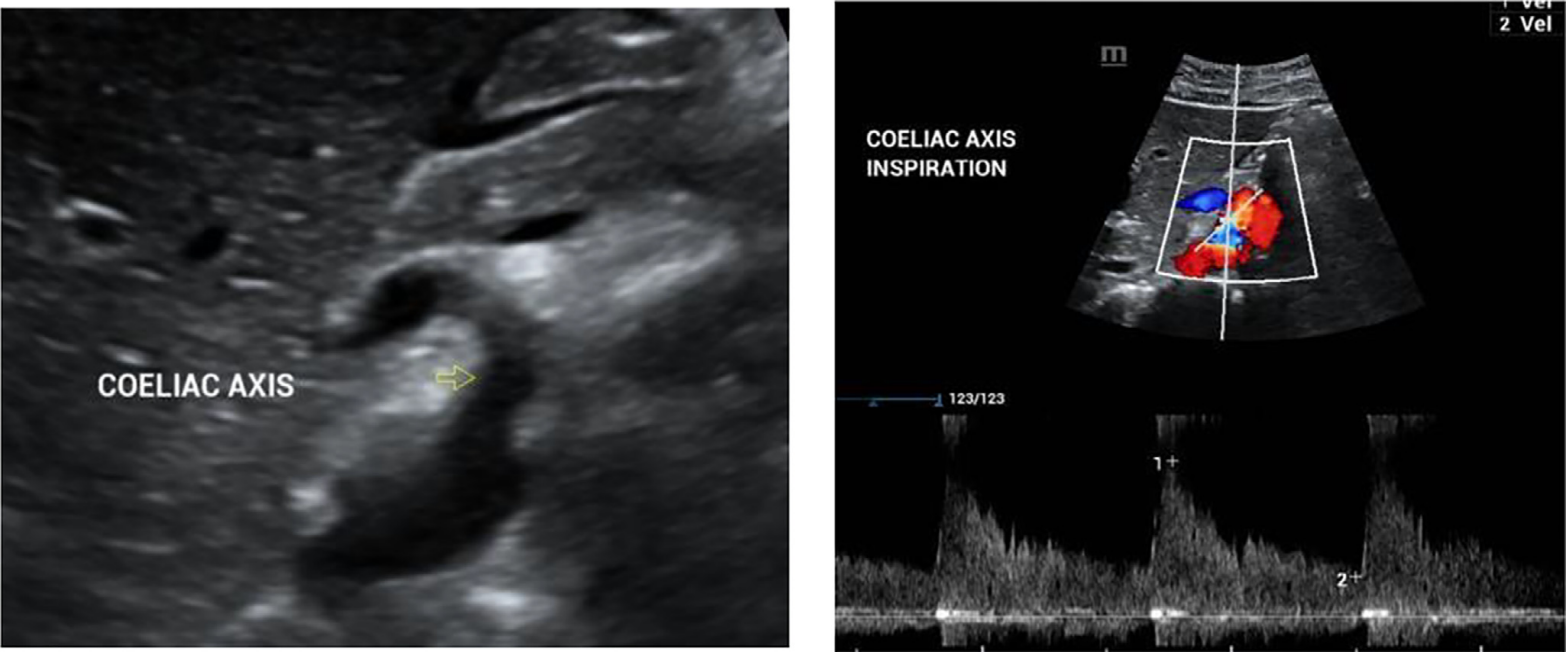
(A): Ultrasound Abdomen image shows the origin of celiac artery from the aorta. (B): Ultrasound Color Doppler with spectral analysis of coeliac artery shows elevated PSV >250 cm/s during inspiration. Note the turbulent flow in the post stenotic segment.
